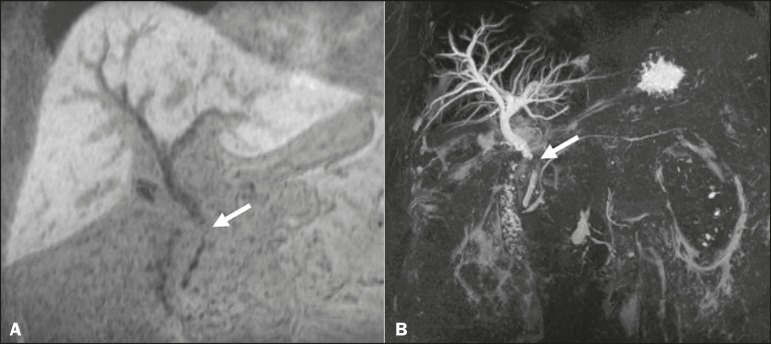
HSCA-enhanced coronal T1-weighted MRC sequence acquired in the late phase
